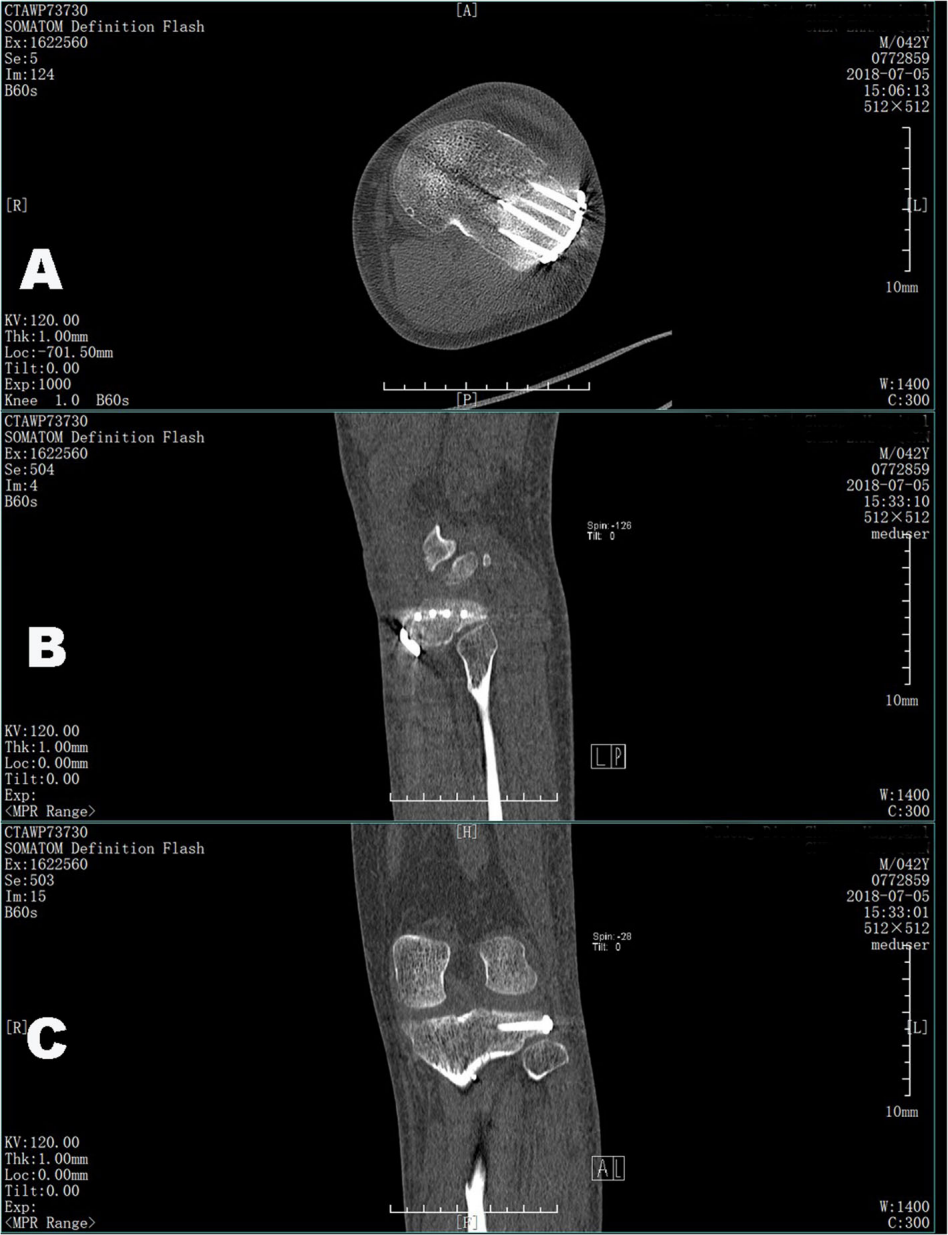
Transverse (A), sagittal (B), and coronal (C) two-dimensional computed tomography (CT) images of the patient in Fig. 3 whose fractured segments were fixed with our plate. At least two rafting screws were used to achieve an adequate grip of the posterolateral quadrant of the tibial plateau (A and B)
